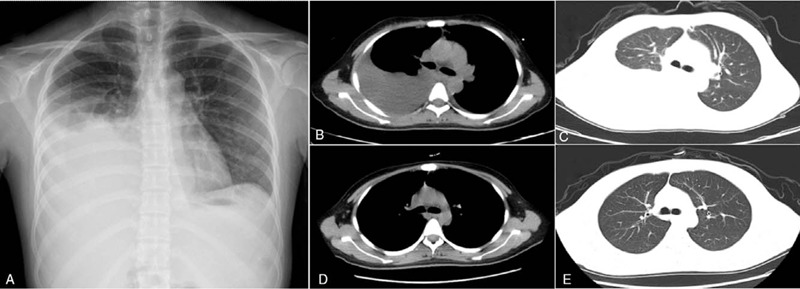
Preoperative and postoperative X-ray and CT findings. Preoperative X-ray of chest showed a massive effusion in the right thoracic cavity (A). Preoperative CT of chest showed a massive effusion in the right thoracic cavity combined with segmental consolidation of the right lower lung (B and C). CT of chest at 5 weeks after surgery showed no abnormal density in either lung or the mediastinum (D and E).
